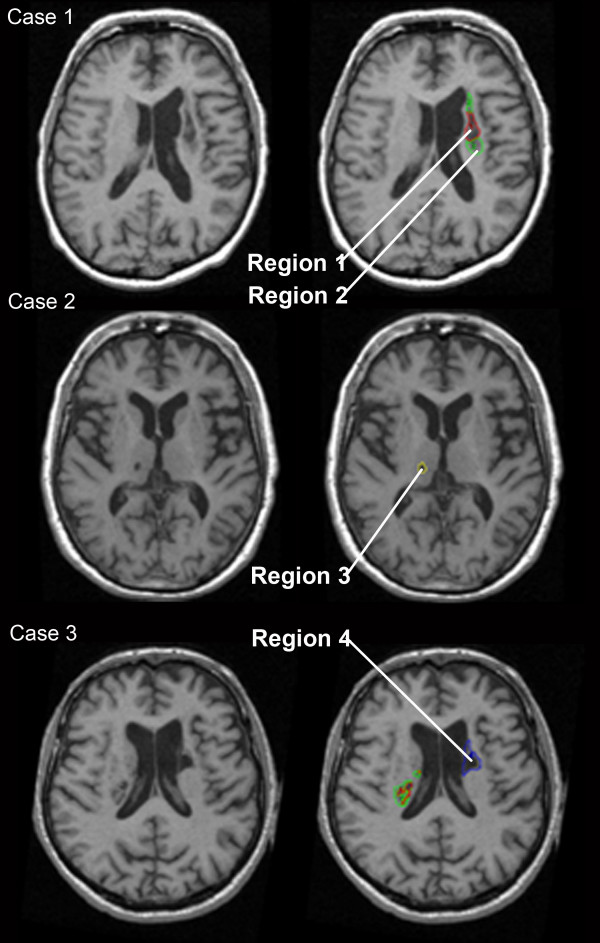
Examples of the four regions used to denote differing categories of lesioned tissue. Note that each row of MR images depicts examples from different patients. Region 1 represents the lesion core, Region 2 represents the peri-necrotic tissue, Region 3 represents lesions in the same hemisphere as the index stroke, and Region 4 represents lesions in the hemisphere opposite to Region 1.
